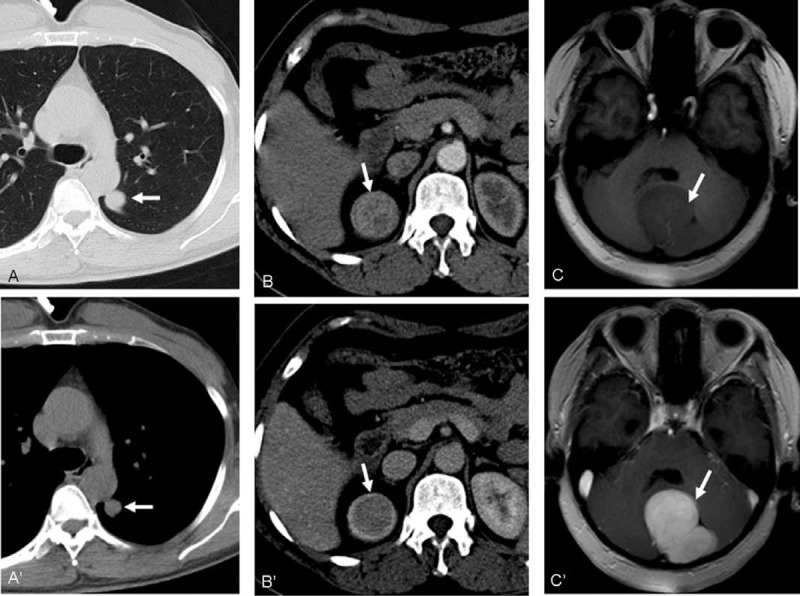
Typical features of small SFTs. A, A’: A 43-year-old man with parenchymal (A) and mediastinal windows (A’) of the CT scan showed a round solitary fibrous tumor of the pleura (≤2.8 × 2.3 cm). B, B’: A 48-year-old female patient presented with a right kidney solitary fibrous tumor. Arterial (B) and portovenous (B’) phases of contrast-enhanced CT showed a spherical, well-defined homogeneous mass with marked homogeneous enhancement. C, C’: A 68-year-old female patient with brain SFT. A T1-weighted axial MRI scan revealed a large lobulated hypointense mass (arrow) in the left cerebellum (C). Gadolinium-enhanced T1-weighted axial MRI (C’) showed marked and homogeneous enhancement of the mass (arrow). CT = computed tomography, MRI = magnetic resonance imaging, SFTs = solitary fibrous tumors.
